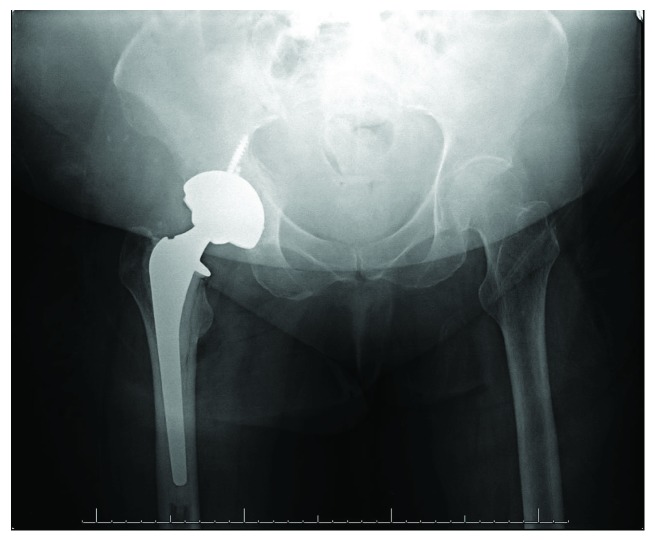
AP pelvis radiograph obtained immediately following conversion to right total hip arthroplasty.
